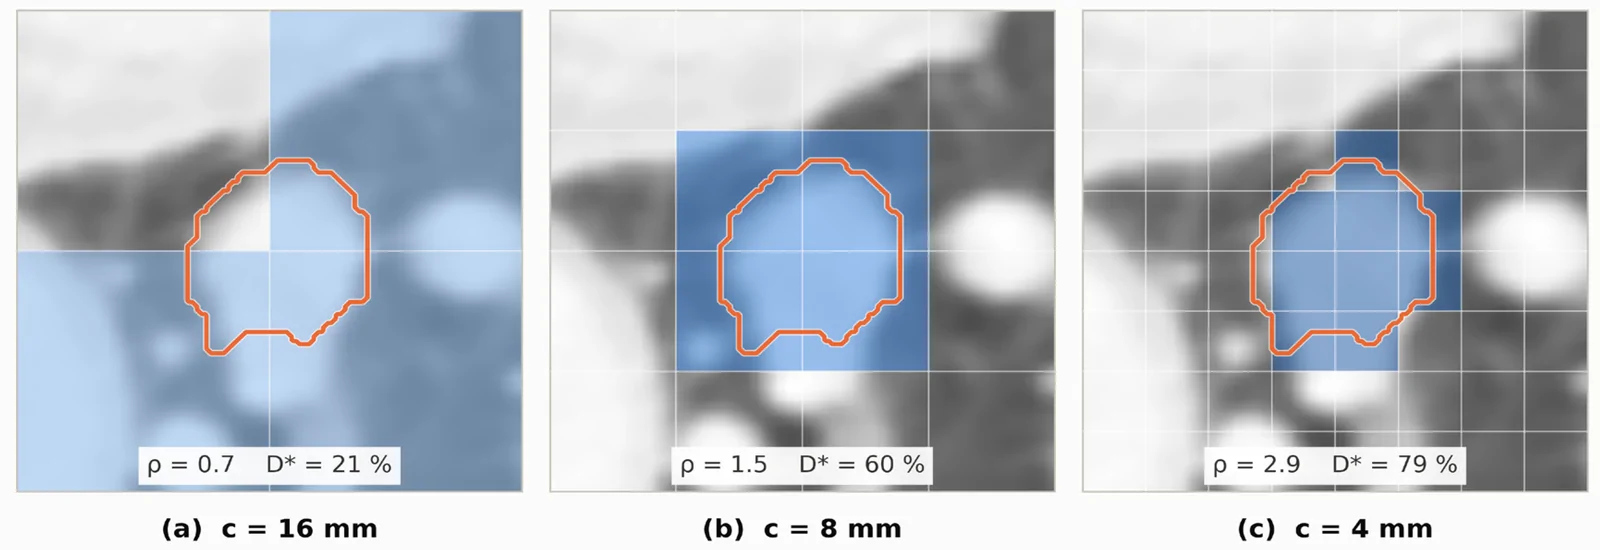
What Equation (7) selects, on the CT. The nodule of Figure 1—13.9 mm nominal, 11.7 mm across the slice shown—in a 32 mm window on three feature grids: (a) c = 16 mm, (b) c = 8 mm, (c) c = 4 mm. Blue is the subset of whole cells maximising Dice against the 50% consensus contour in orange, which is the best prediction any classifier deciding on that grid could produce. Values are for this single grid offset, whereas Table 2 averages over the offset lattice of Equation (8).
